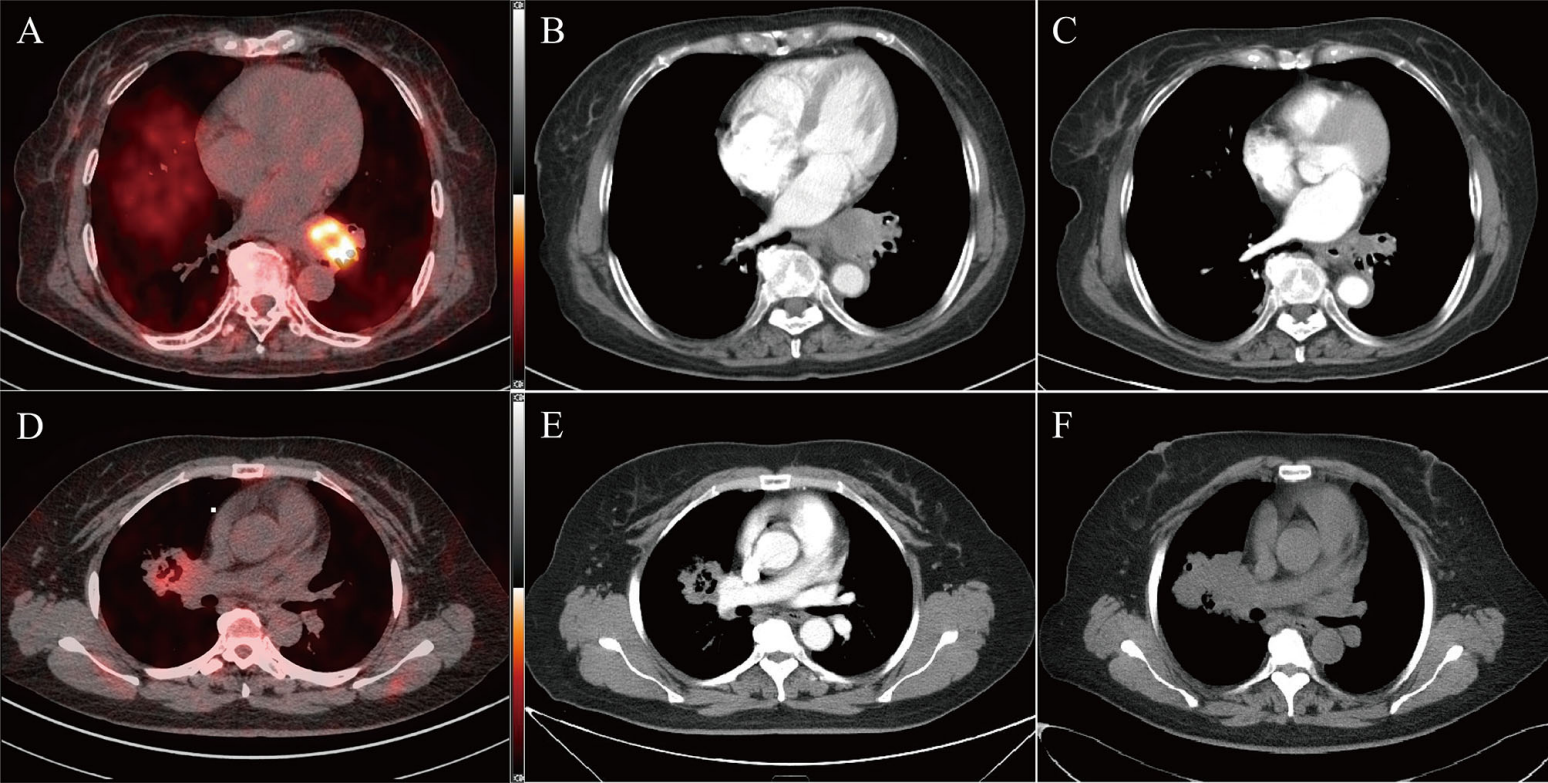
Two typical examples of ^18^F-RGD PET/CT scans in responder (top panel, SUVmax = 7.99, SUVmean = 3.39, MTV = 5.02, Response evaluated as PR) and non-responder (bottom panel, SUVmax = 3.38, SUVmean = 2.76, MTV = 2.07, Response evaluated as PD). Baseline PET/CT images (A, D) and corresponding CT slices from before treatment (B, E) and 2 cycles after treatment (C, F).
